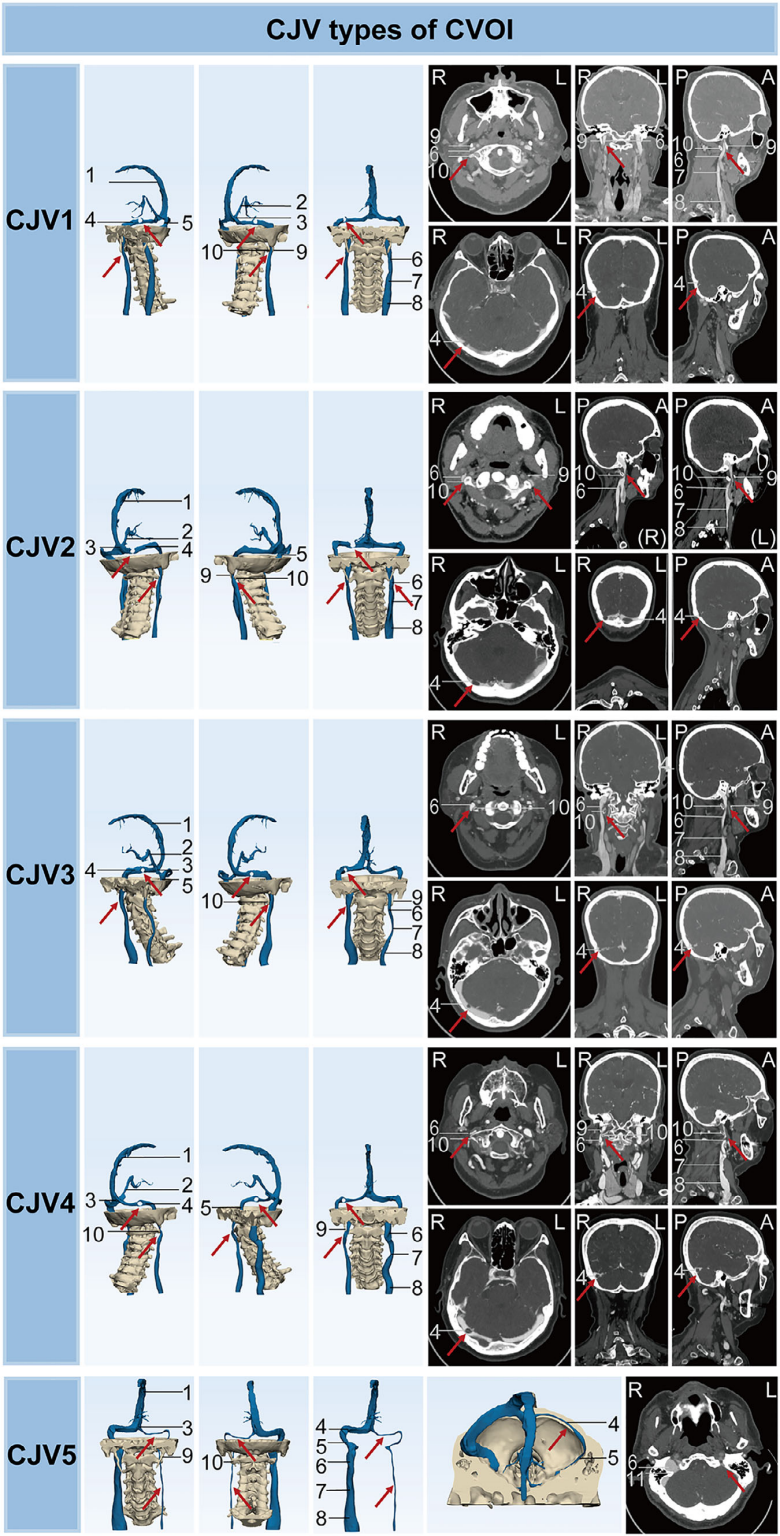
Imaging characteristics of CJV types of CVOI on CT. Numbered labels: 1 = superior sagittal sinus, 2 = inferior sagittal sinus, 3 = confluence of sinuses, 4 = transverse sinus, 5 = sigmoid sinus, 6 = internal jugular vein (J3 segment), 7 = internal jugular vein (J2 segment), 8 = internal jugular vein (J1 segment), 9 = styloid process, 10 = transverse process of the atlas (C1), 11 = jugular foramen. CVOI, cerebral venous outflow insufficiency; CJV, intracranial and extracranial tandem type of CVOI; R, right; L, left; P, posterior; A, anterior.
